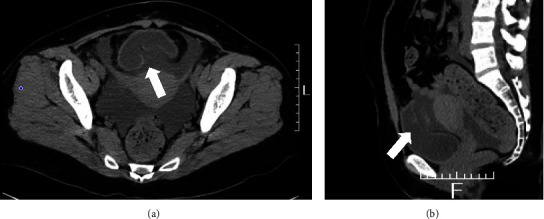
Computed tomography images of the bladder show a rupture from the posterior wall: (a) axial view; (b) sagittal view. Arrows point to the ruptured wall.
